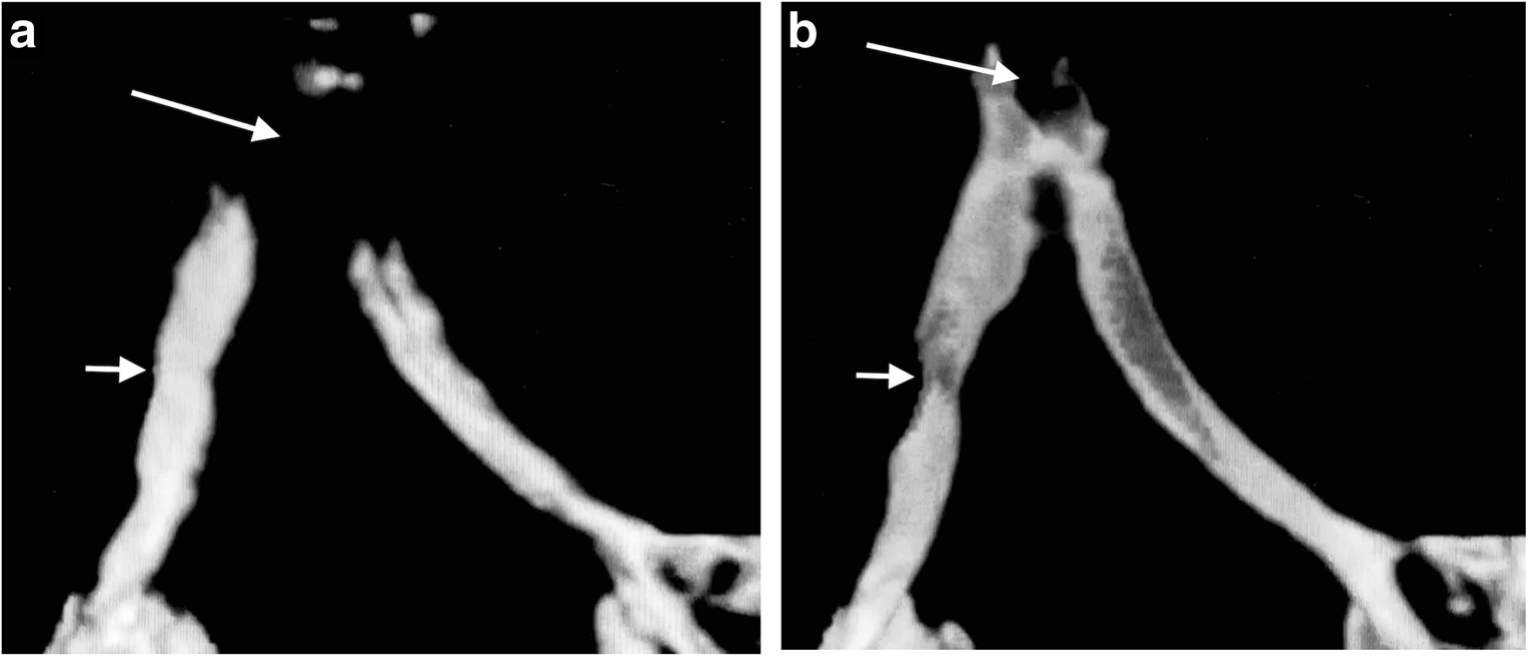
Frontal views of a dynamic 4-D CT performed in a 6-month-old girl with bronchomalacia involving the bronchus intermedius. a, b Frontal views of the volume-rendered reconstructions of two out of four phases of a dynamic 4-D CT study of the airways demonstrate an artefact arising from the endotracheal tube tip positioned at the carina (*long arrows*), which precludes evaluation of the trachea. The tube position changes from inspiratory phase (a) to the expiratory phase (b), which demonstrates the bronchomalacia involving the bronchus intermedius (*short arrows*). Despite demonstrating the bronchomalacia, this study was recorded as nondiagnostic because it failed to evaluate the trachea
